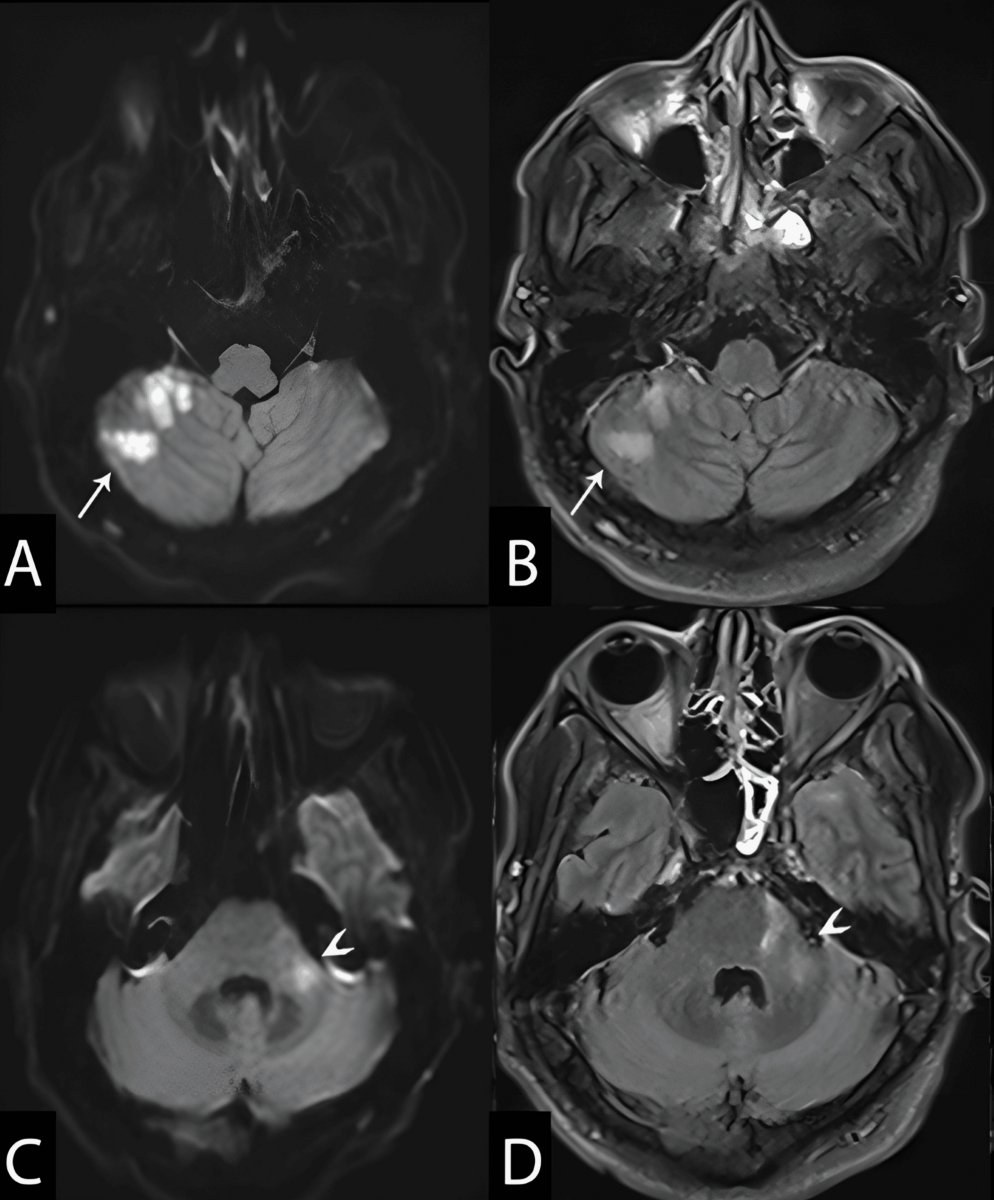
Brain MRI on day 48 All images are in the axial plane. Panels A and C show DWI b1000 images; panels B and D show T2 FLAIR images. The arrow indicates an acute ischemic infarction in the right cerebellar hemisphere, and the arrowhead marks an early subacute infarction in the left middle cerebellar peduncle. DWI, diffusion-weighted imaging; FLAIR, fluid-attenuated inversion recovery
